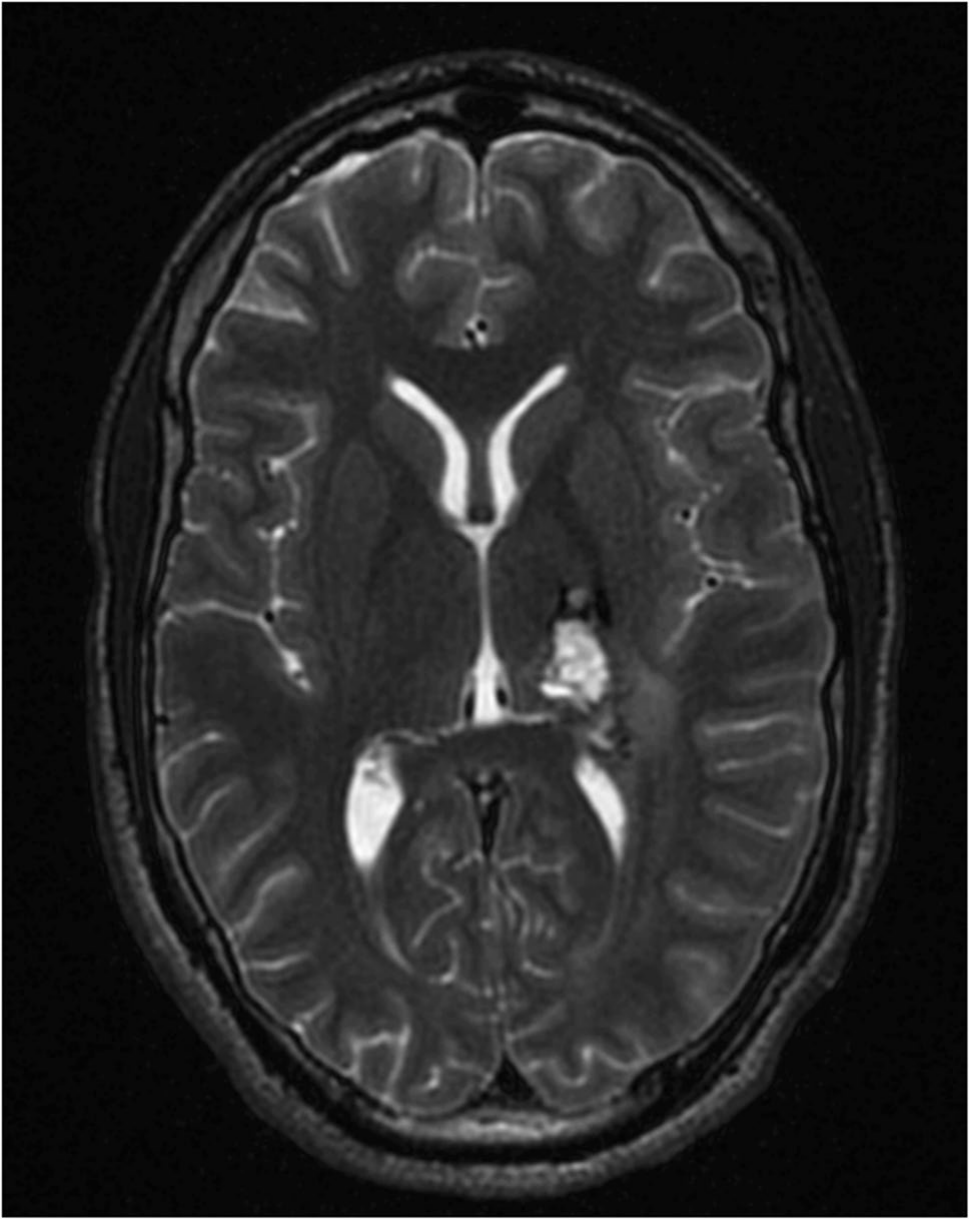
Axial T2-weighted magnetic resonance imaging showing complete resection of cavernoma
